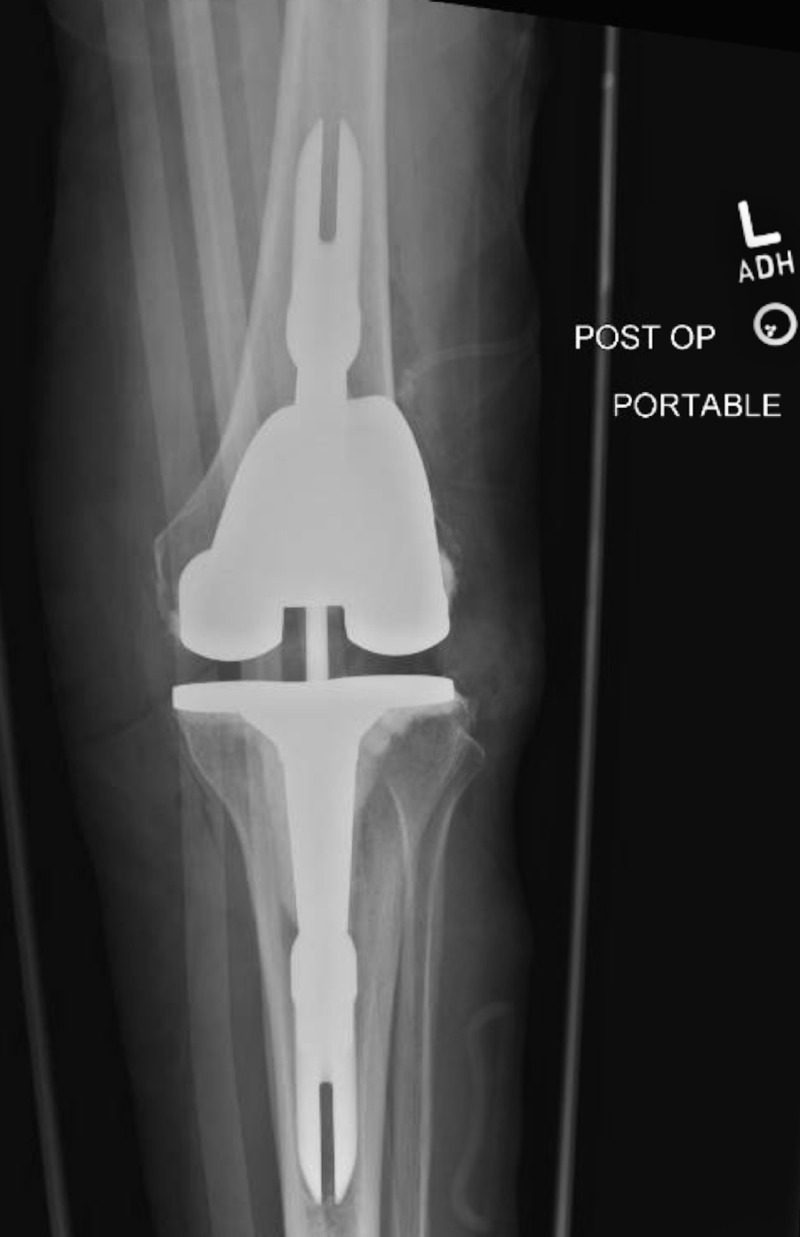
Postoperative anteroposterior radiograph confirming proper placement of left revision total knee arthroplasty (TKA) components
